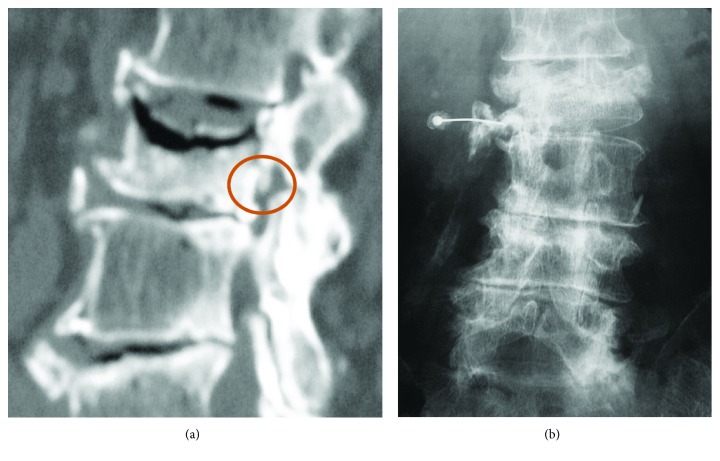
(a, b) Three-dimensional computed tomography image showing the bone tip under the right L2 pedicle. Left L2 root block was effective temporally, and radiography showed that left L2 root compressed at intervertebral foramen.
